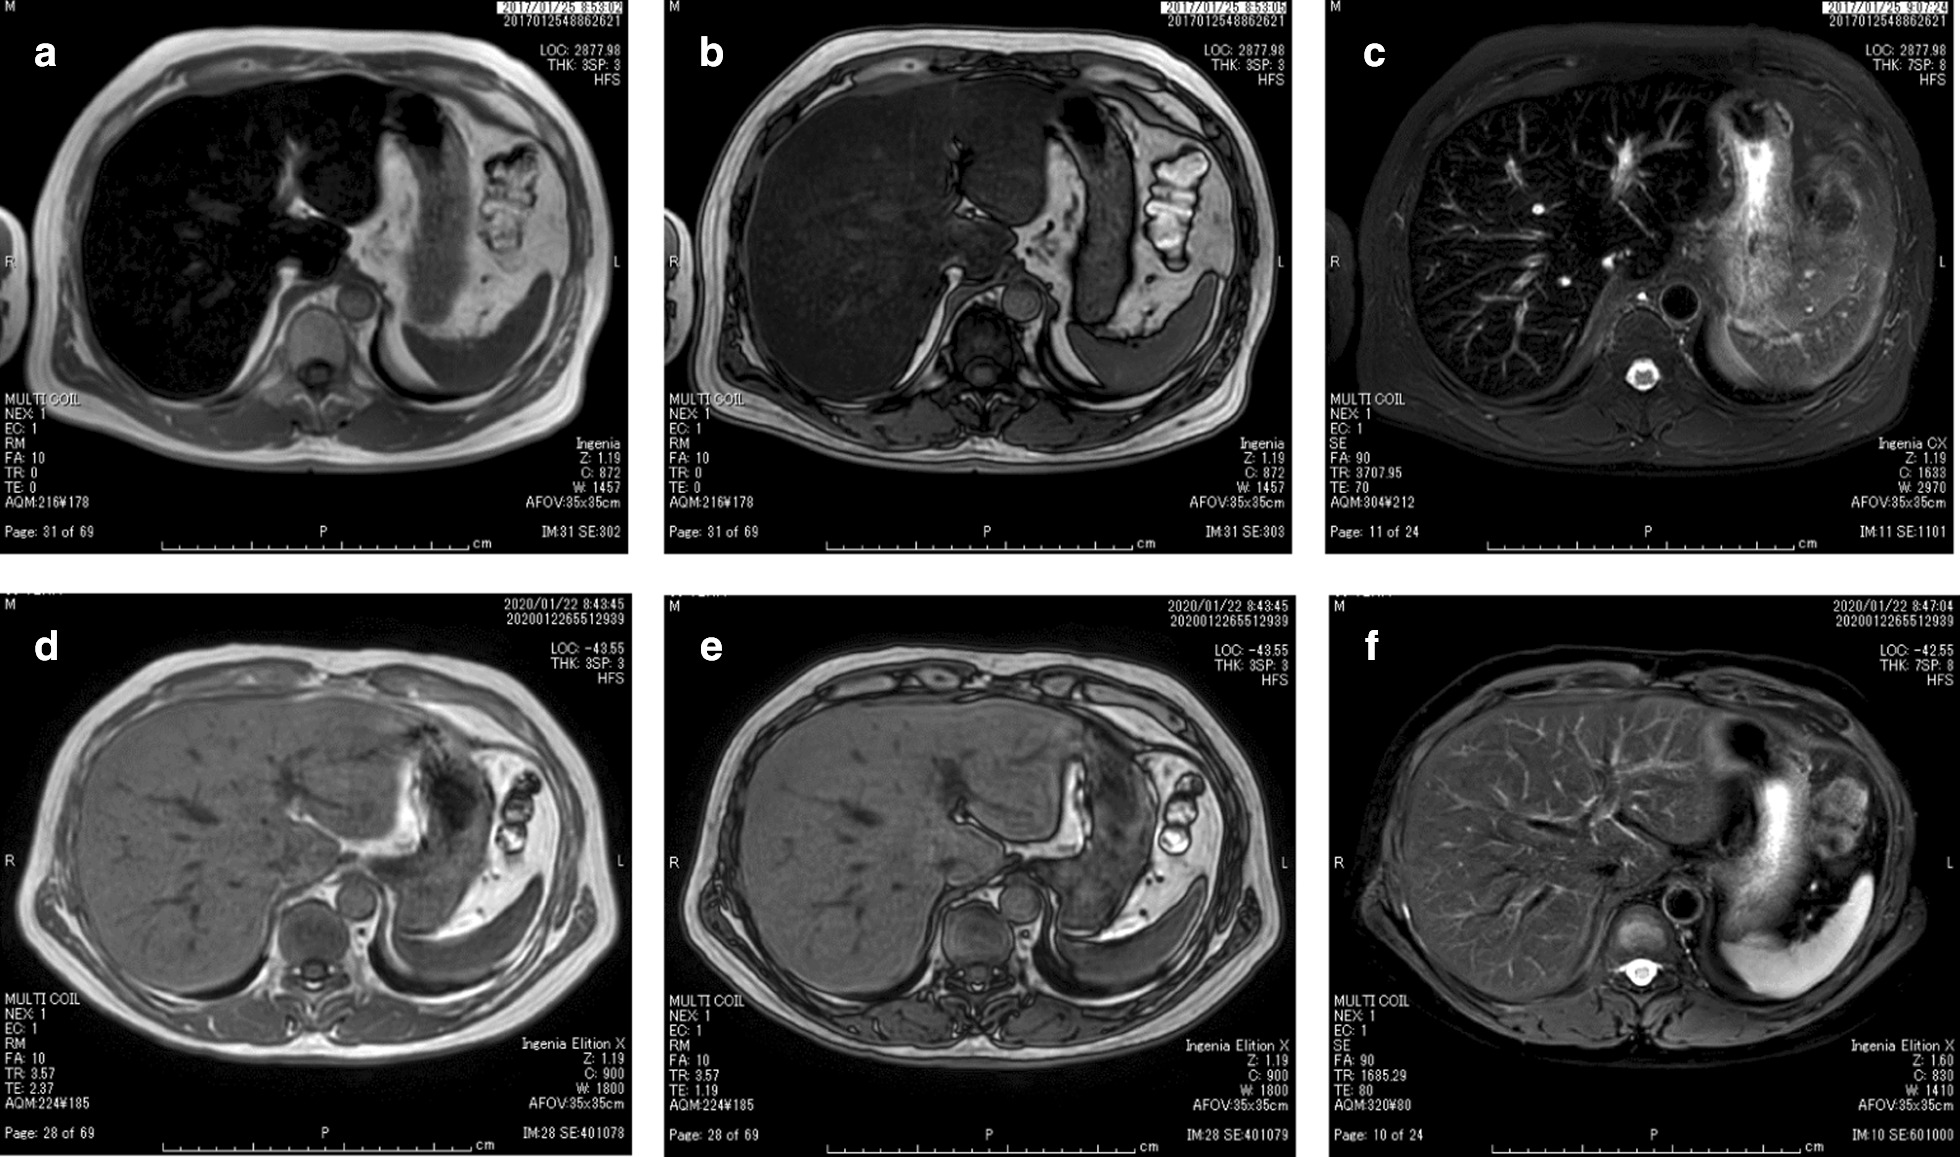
Magnetic resonance imaging of the liver before (2017/01) and after (2020/01) phlebotomy. a T1-weighted in-phase image before phlebotomy. b T1-weighted out-of-phase image before phlebotomy. c T2-weighted image before phlebotomy. d T1-weighted in-phase image after phlebotomy. e T1-weighted out-of-phase image after phlebotomy. f T2-weighted image after phlebotomy
